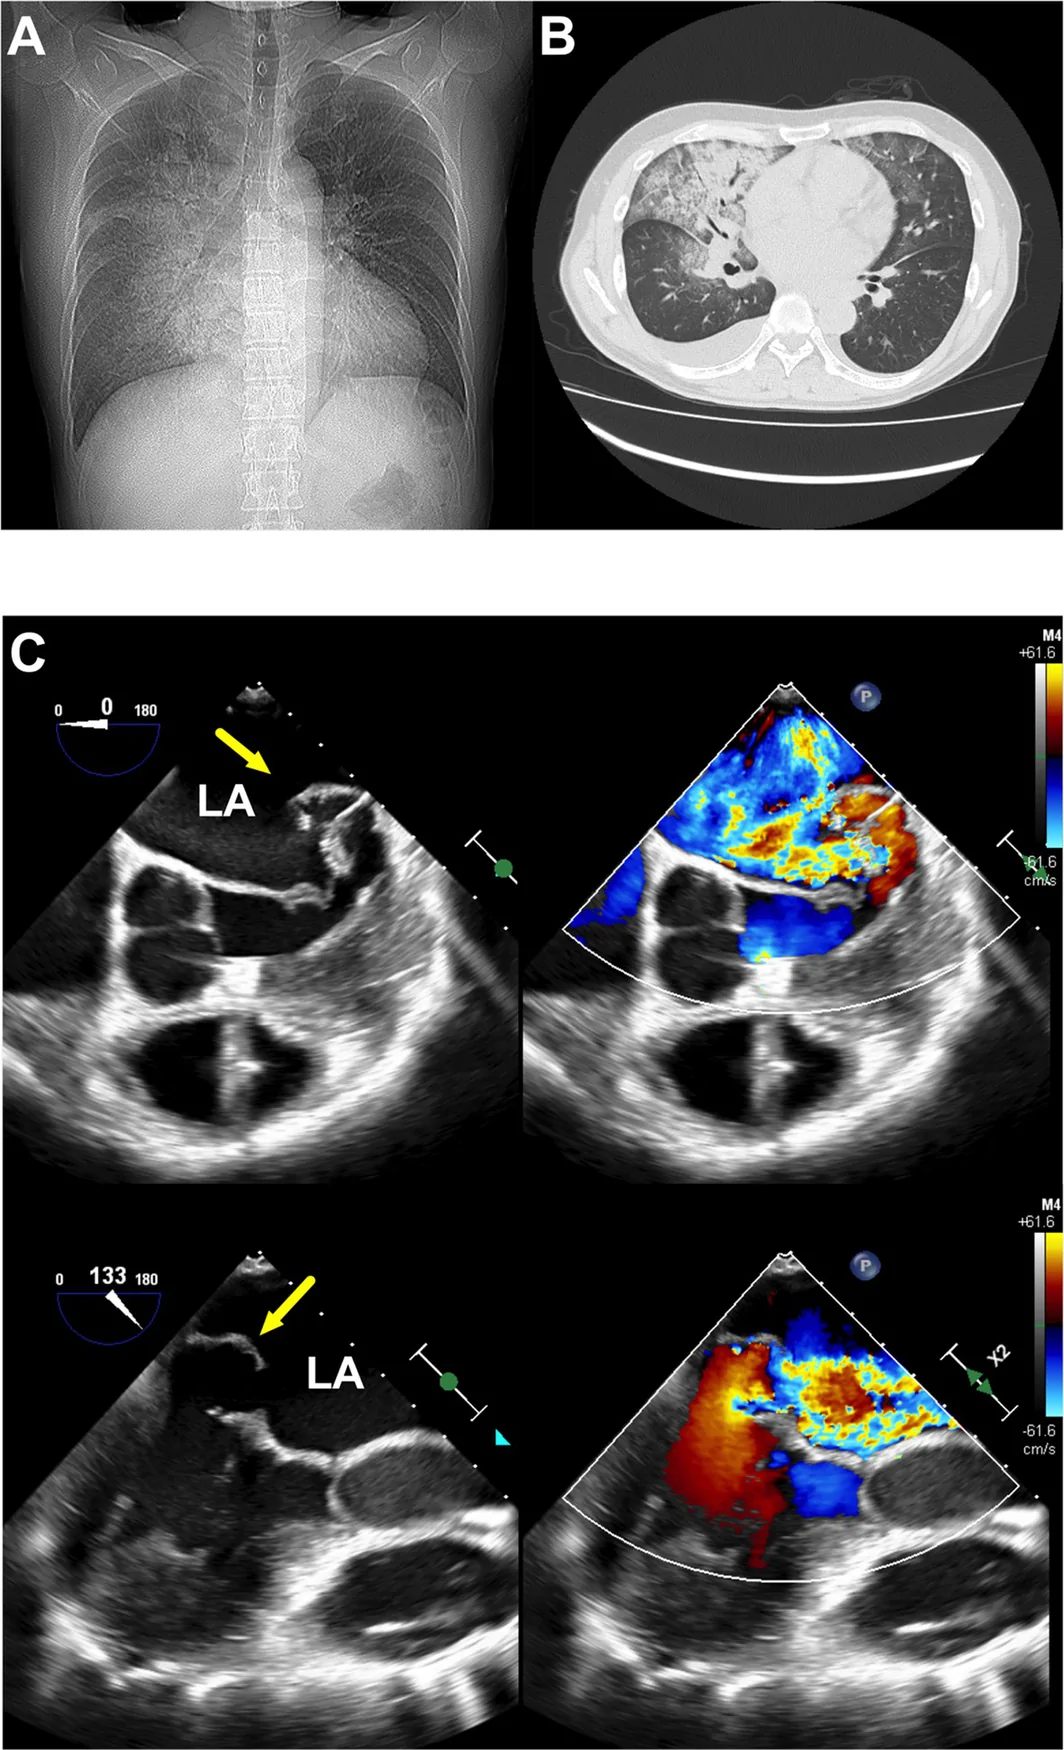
Mitral valve prolapse mimicking pneumonia on clinical imaging. (A) Chest radiography showed increased vascular markings with pulmonary infiltration. (B) Computed tomography demonstrated ground‐glass opacities with infiltrative changes and pleural effusion, predominantly in the right lung field. (C) Transesophageal echocardiography (Left) and Color Doppler images (Right) revealed mitral valve prolapse with eccentric mitral regurgitation, demonstrating a strong turbulent flow signal directed toward the left atrium. LA indicated the Left Atrium, and the yellow arrow showed the prolapsed mitral valve.
